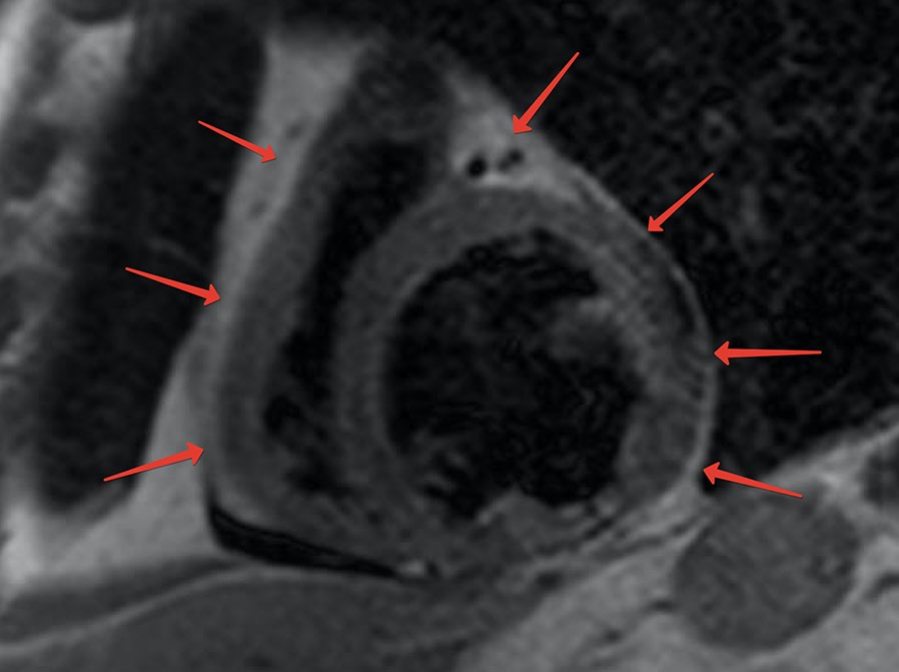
Epicardial fat (red arrows) on the MRI of the heart on a short axis
